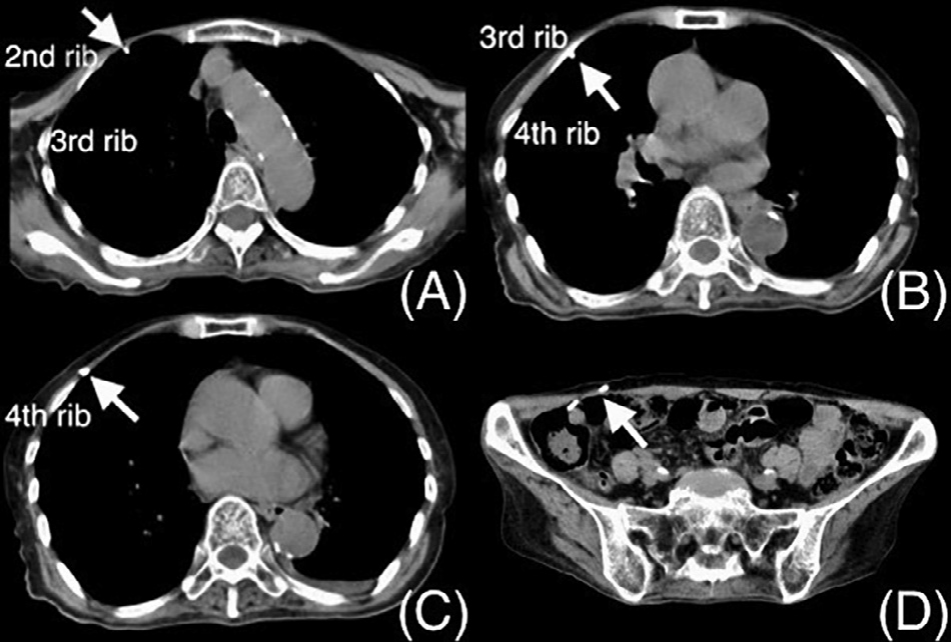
Chest and abdominal computed tomography scans taken 13 days after the first ventriculoperitoneal shunt procedure showing the distal catheter (white arrows) passing only under the second rib (A) and third rib (B), and over the fourth rib in the subcutaneous layer (C), and in the abdominal space (D).
